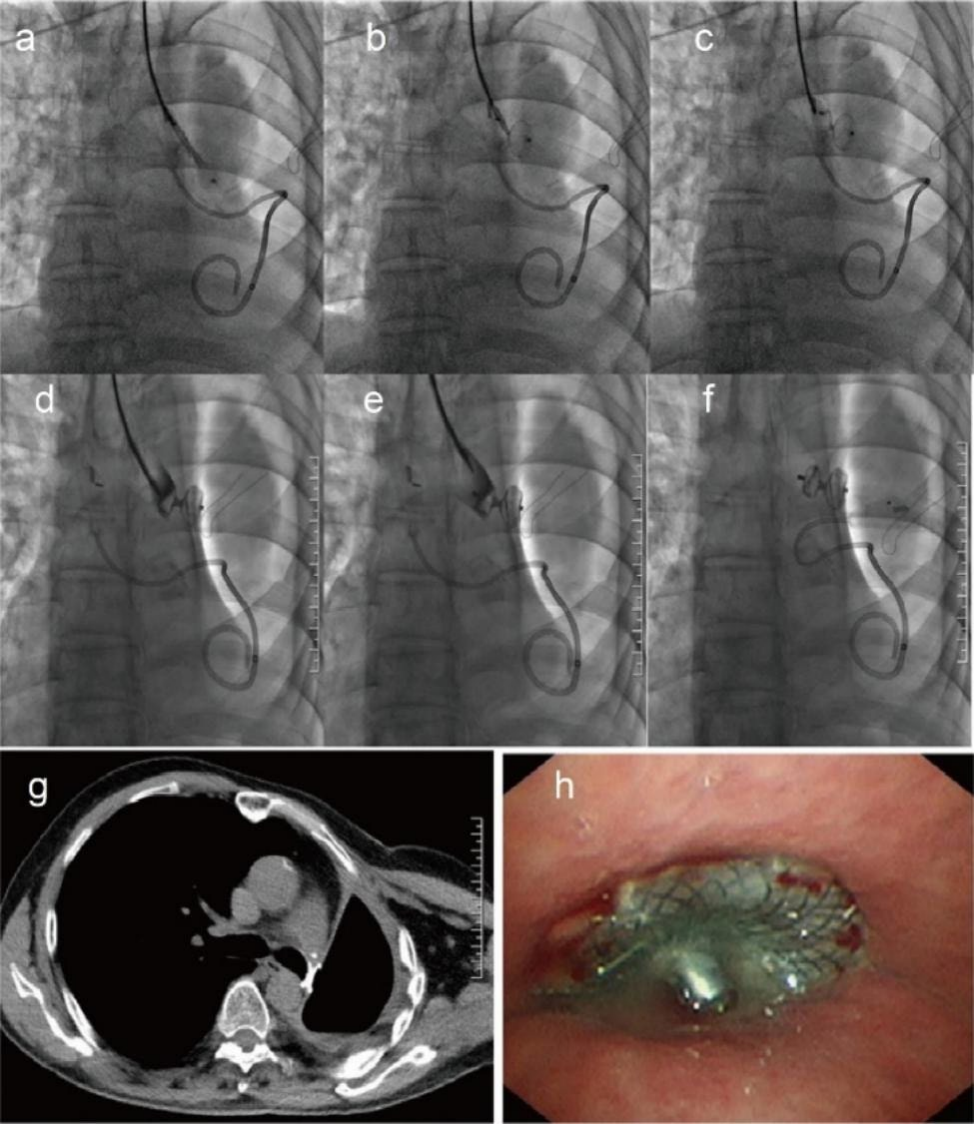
The process of VSD placement and follow-up. After the bronchogram, the sheath containing the VSD was introduced into the BPF. First, the distal disk was released (a) and pulled back to occlude the fistula (b). Then, the proximal disk was released to anchor the residual bronchus (c). The bronchogram via a catheter demonstrates the successful occlusion of BPF (d). The VSD was detached (e) and left in place to occlude the BPF (f). Representative images of VSD-occluded BPF from CT (g) and bronchoscopy (h)
